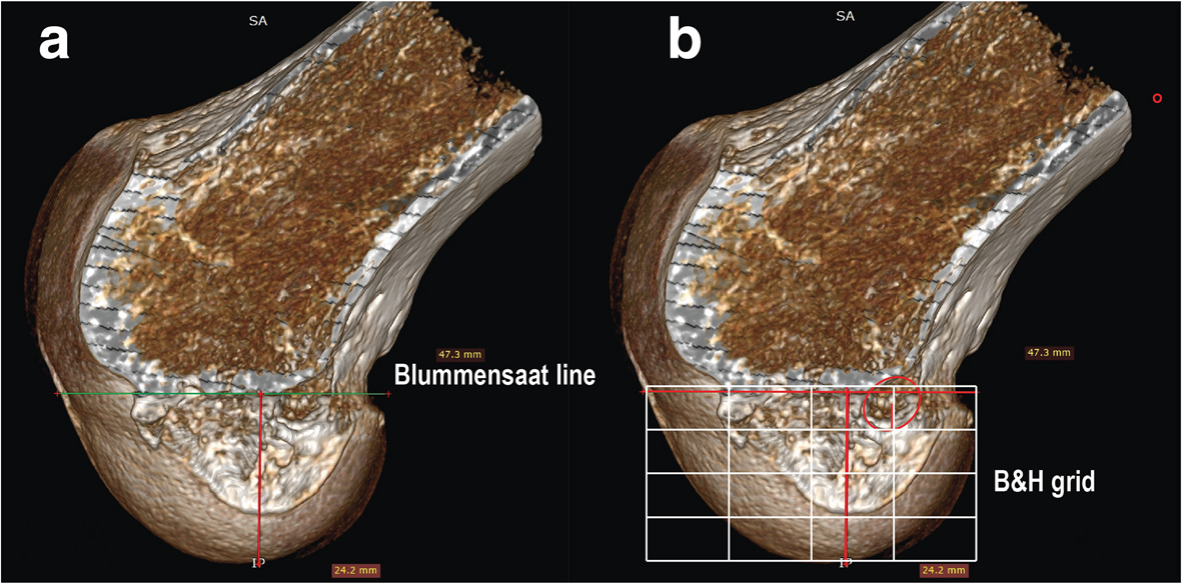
a 3D CT-scan reconstruction of the lateral femoral condyle. Length and width of the two axes of the construction of Bernard & Hertel quadrant method are measured. b Example of tunnel center position measures using Bernard & Hertel quadrant method on the 3D CT-scan reconstruction
